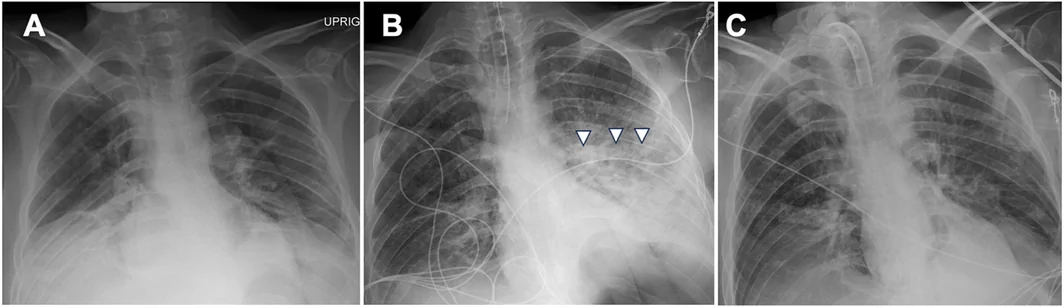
Serial chest radiographs showing the progression and subsequent improvement of bilateral pulmonary opacities and left‐predominant consolidation following tigecycline discontinuation, cefiderocol initiation and corticosteroid therapy. (A) Six days before tigecycline initiation, showing mild bilateral hazy pulmonary opacities. (B) On Day 21 of tigecycline therapy, demonstrating new dense left‐predominant consolidation, as indicated by white arrowheads. (C) The patient's respiratory status and imaging findings improved progressively over the following weeks, with radiographic improvement. He was discharged to a long‐term acute care hospital on 30 January for continued respiratory care and rehabilitation. The complete clinical course is summarised in Figure 3.
